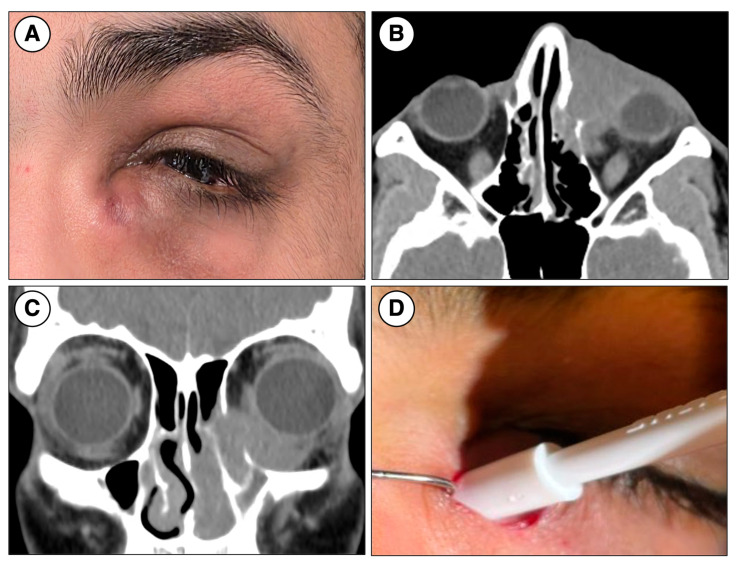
(A) A firm and painless left medial canthal mass, extending into the lower eyelid and above the canthal tendon, was evident in a 32-year-old patient with 4 months of hemolacrima. (B,C) A relatively homogeneous hypodense lesion, centered on the lacrimal drainage system, was demonstrated on CT, together with bone erosion and extension into the medial orbit and sinonasal cavity. (D) Transcutaneous punch biopsy confirmed diffuse large B-cell lymphoma.
